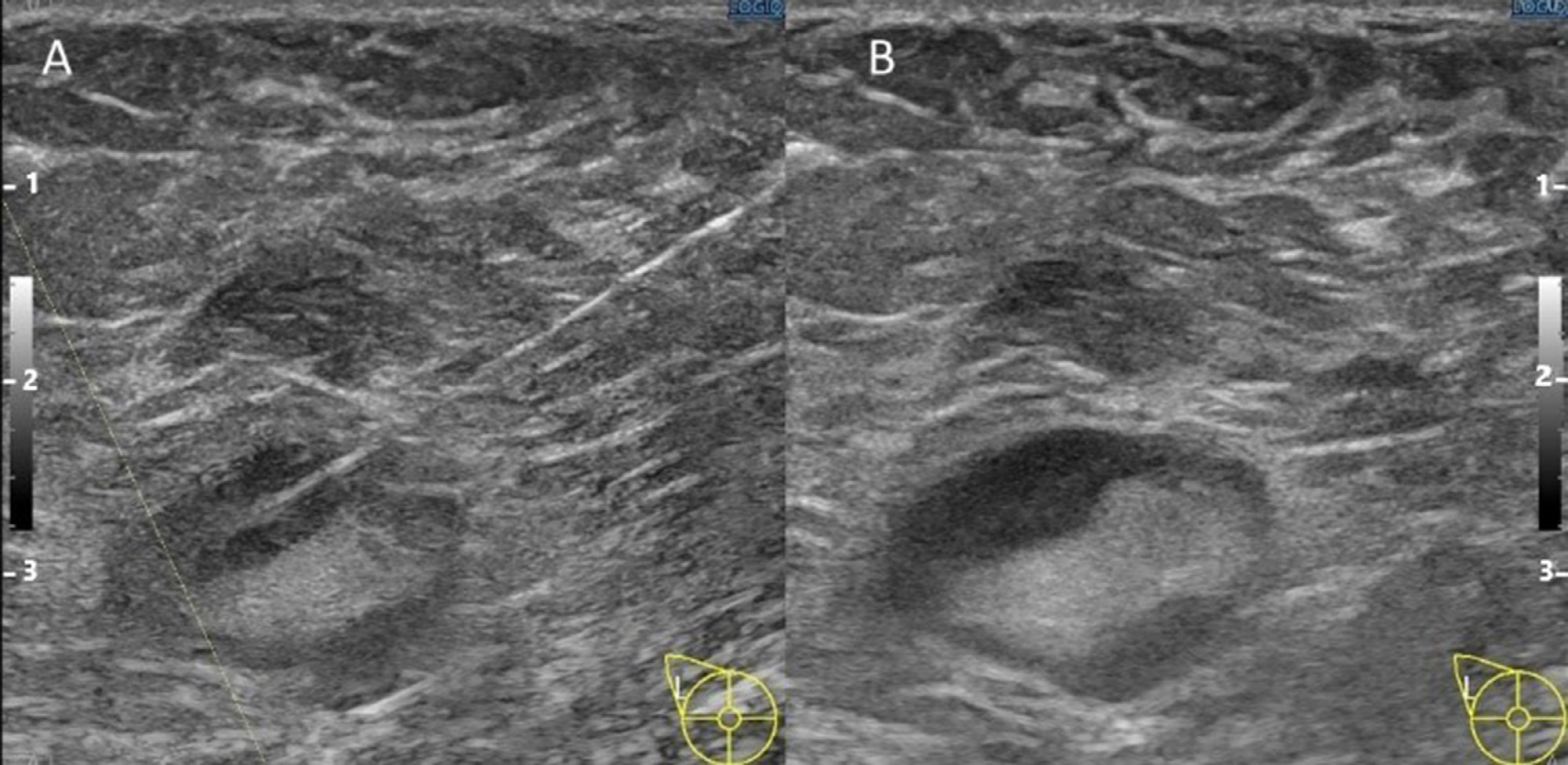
Ultrasound-guided fine-needle (23G) aspiration for left axillary lymph node. Combination of “Survey mode” and “B-Steer+” (A) and normal mode (B). The position of the needle is the same in both images, and the ultrasound mode is switched. The axillary lymph nodes in Figure 5 and Figure 3B are the same. In the normal mode, the visibility of the biopsy needle was poor. However, with the addition of “Survey Mode” and “B-Steer+” the visibility of the needle improved.
